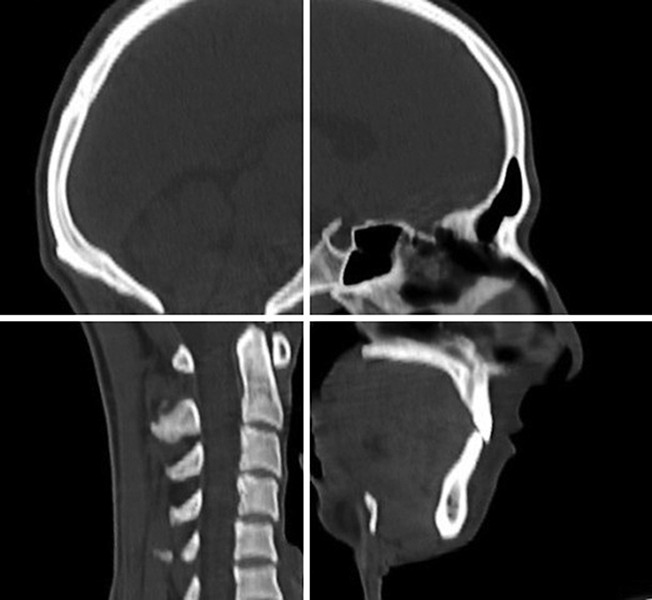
Definition of the spatial coordinate system for the three-dimensional cone beam computed tomography images. The Frankfort horizontal plane and the sagittal plane
